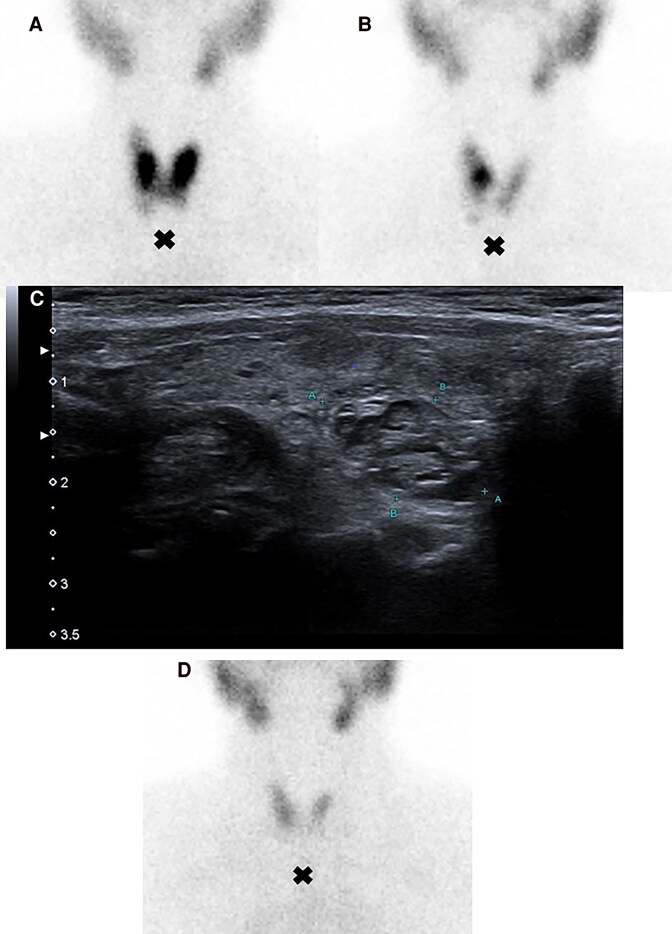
Cross indicates the patient' suprasternal notch. (A) 24-hour thyroid radioactive iodine test revealed high uptake (57%; normal reference: 8%-32%) and a diffuse distribution, strengthening the diagnosis of Graves disease. (B) A 24-hour thyroid radioactive iodine test showed high uptake of 36% and a solitary “hot” thyroid nodule in the right lobe, supporting the diagnosis of solitary toxic thyroid nodule. (C) Thyroid ultrasound revealed 1 cystic-solid isoechoic nodule in the right lobe, measuring 1.8 × 1.0 × 0.9 cm, reinforcing the diagnosis of toxic thyroid nodule. (D) A 24-hour thyroid radioactive iodine scan after iodine-131 treatment with 1.11 MBq (30 mCi) showed a homogeneous diffuse thyroid distribution, demonstrating a successful treatment of the solitary toxic thyroid nodule.
